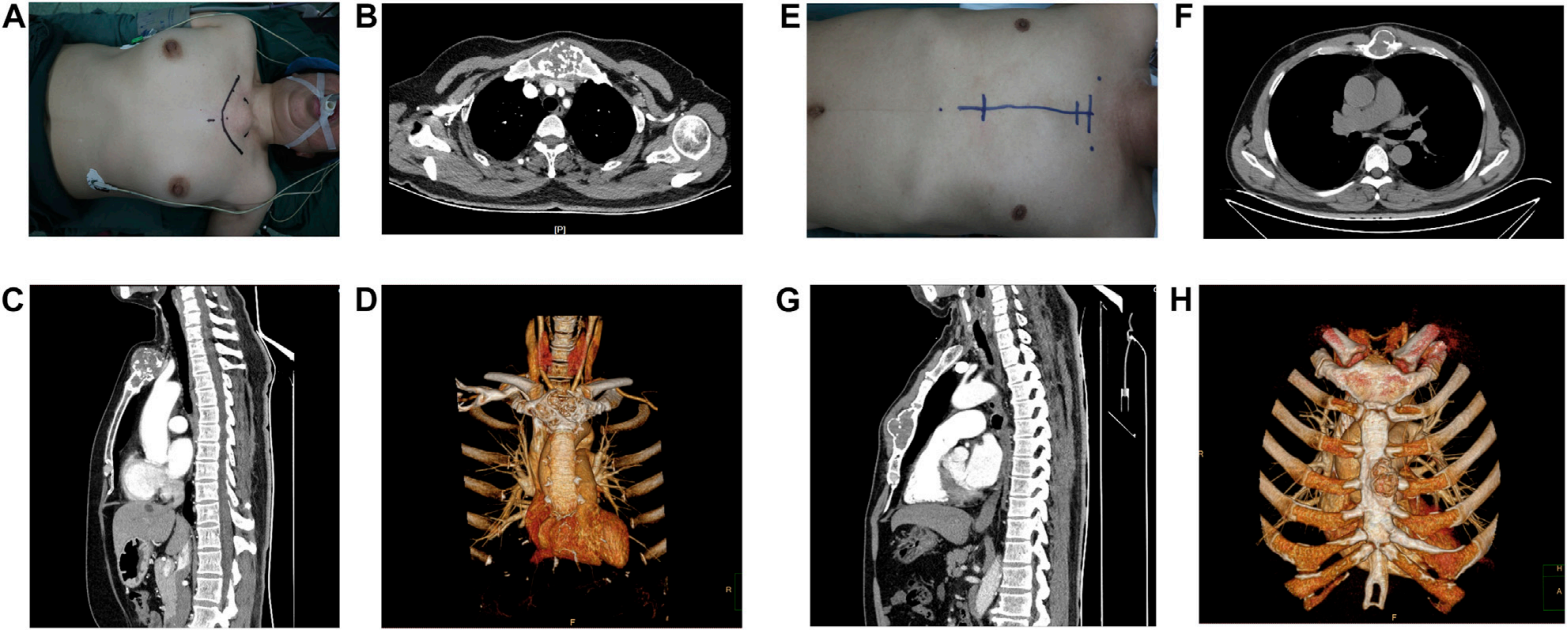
Appearance and preoperative images of the patients. (A–D) Female patient and (E–H) Male patient. (A,E) Appearance of the lesion and operative route on the body surface. (B,C) (F,G) The tumour is shown in cross-sectional and sagittal views (D,H), (D) reconstruction of tumour on CT is demonstrated.
